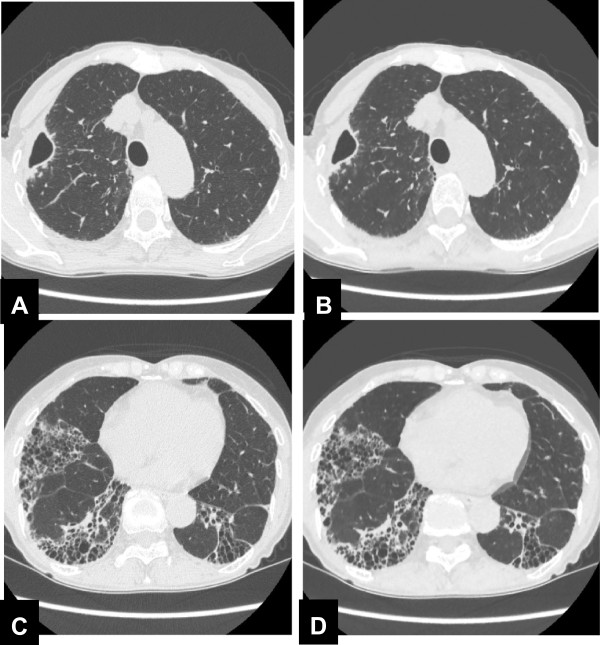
Standard-dose FBP CT images at the level of aortic arch (A) and at the level of lower lung lobe (C), and low-dose MBIR CT images at the corresponding level (B and D) in a 67 year-old woman with lung cavities and reticular opacity. Image quality score for those lesions on low-dose MBIR CT are both graded 5, showing equivalent on standard-dose FBP CT. Objective image noise measured is 13.04 on standard-dose FBP CT and 11.09 on low-dose MBIR CT in this patient.
